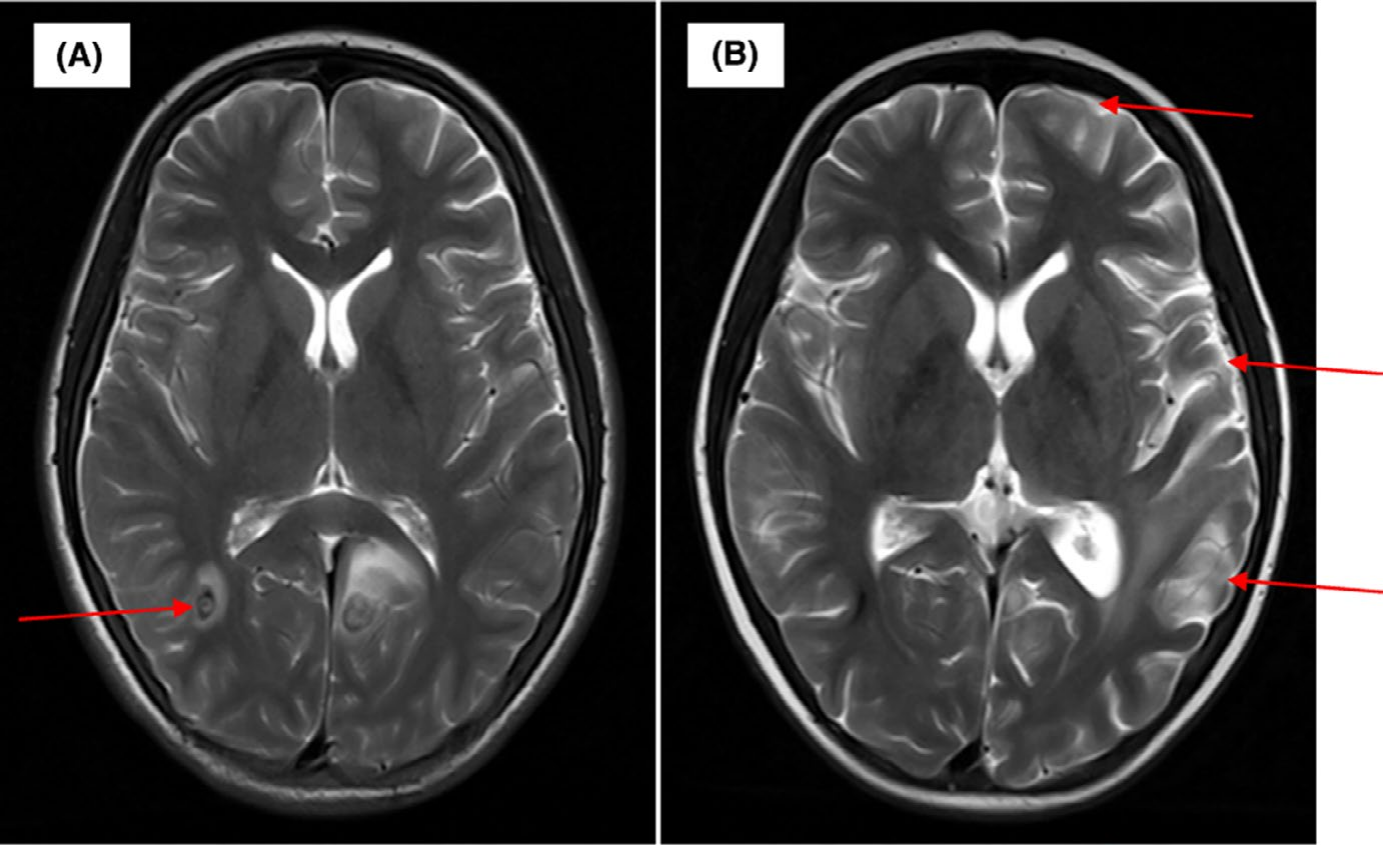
Brain MRI before chemotherapy showed extensive infiltration of the dura mater and hemorrhage of the right parietal lobe and bilateral occipital lobe on T2‐weighted imaging (A) and the MRI showed encephalopathy of the left parietal lobe, occipital lobe and temporal lobe and decreased infiltration of the dura mater on T2‐weighted imaging during chemotherapy(B)
